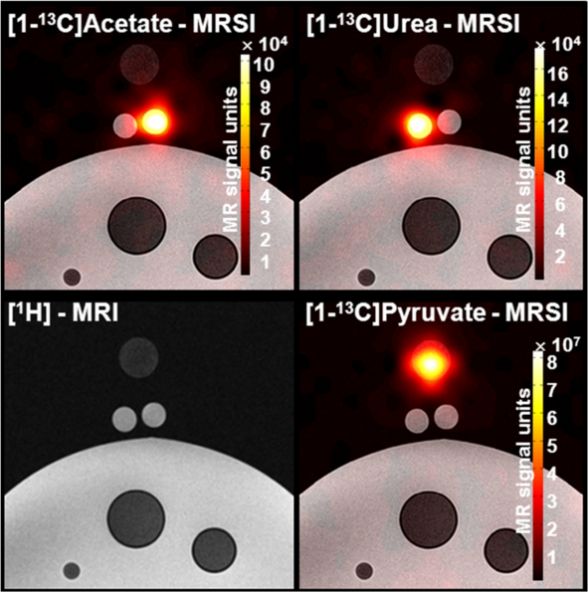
Examples of ^13^C-MRSI. ^13^C-MRSI metabolite images (*color*, *scale bar inserted*) overlaid on ^1^H-MRI (*lower left*). *upper row*: Acetate and urea metabolite images. *lower right*: Image of hyperpolarized pyruvate
